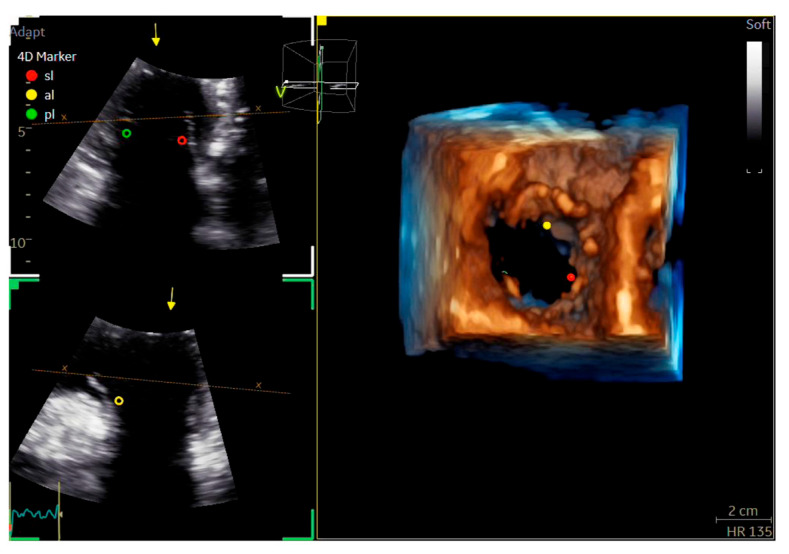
Example of automated identification of the tricuspid valve leaflets in three-dimensional viewing in echocardiography.
